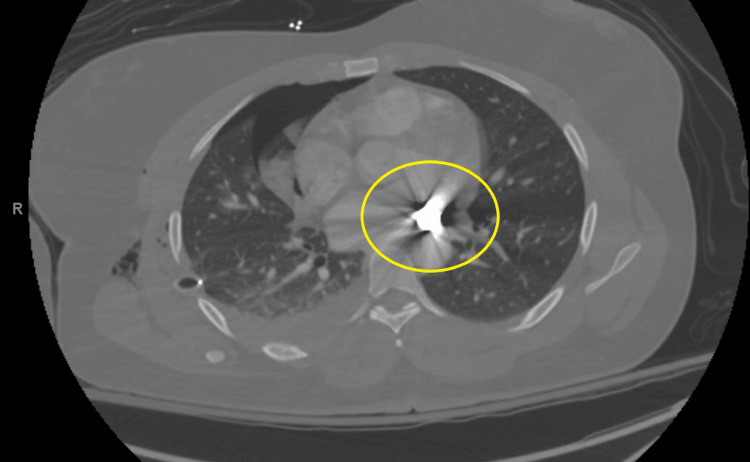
Preoperative CTA A hyperdense object was identified adjacent to the left inferior pulmonary vein.
